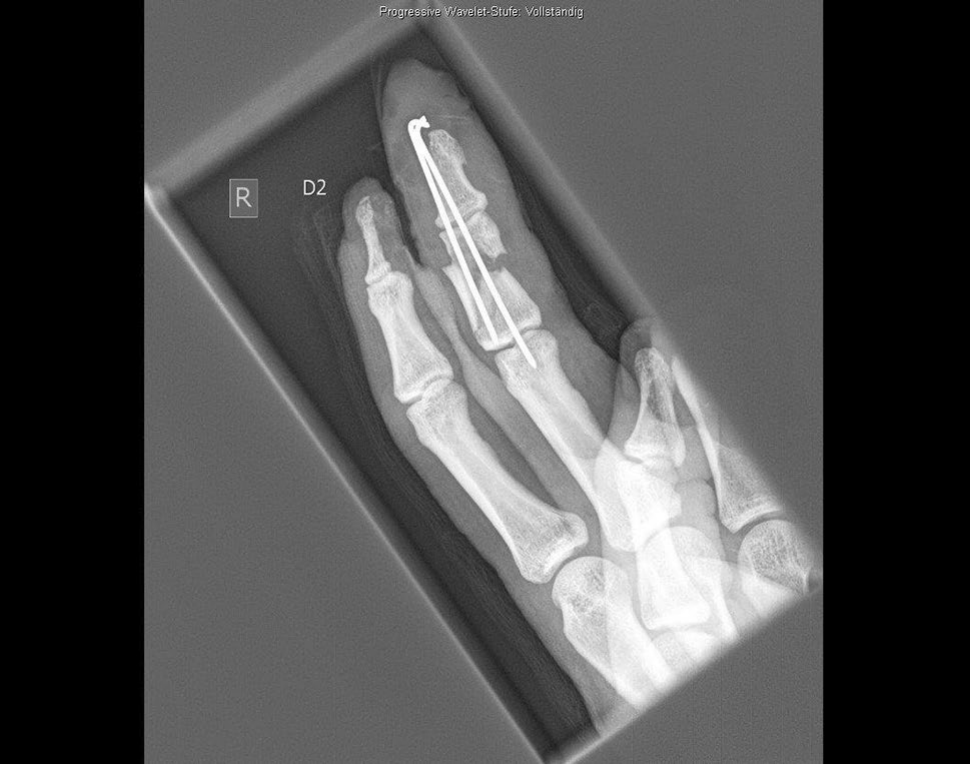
Case Presentation 2: Postoperative X-ray after the first operation
